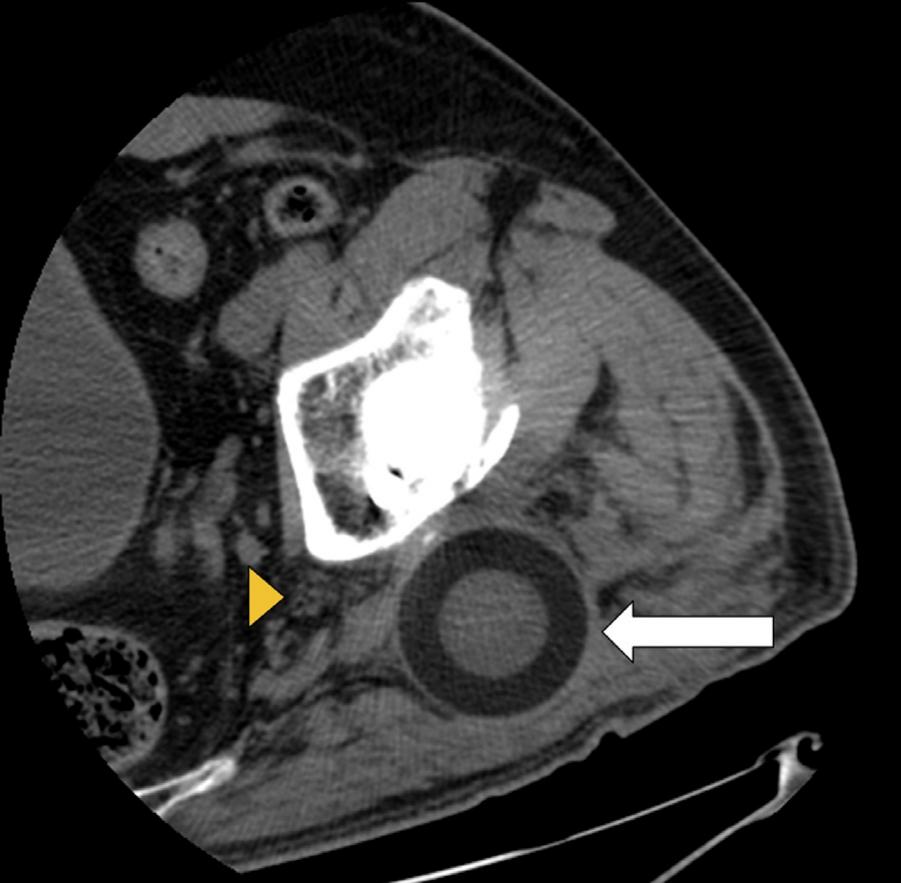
Axial CT image also shows that the polyethylene head (arrow) is displaced in the posterior gluteal musculature. The displaced head does not definitively contact the sciatic nerve (arrowhead) on this CT obtained with the patient lying supine.
